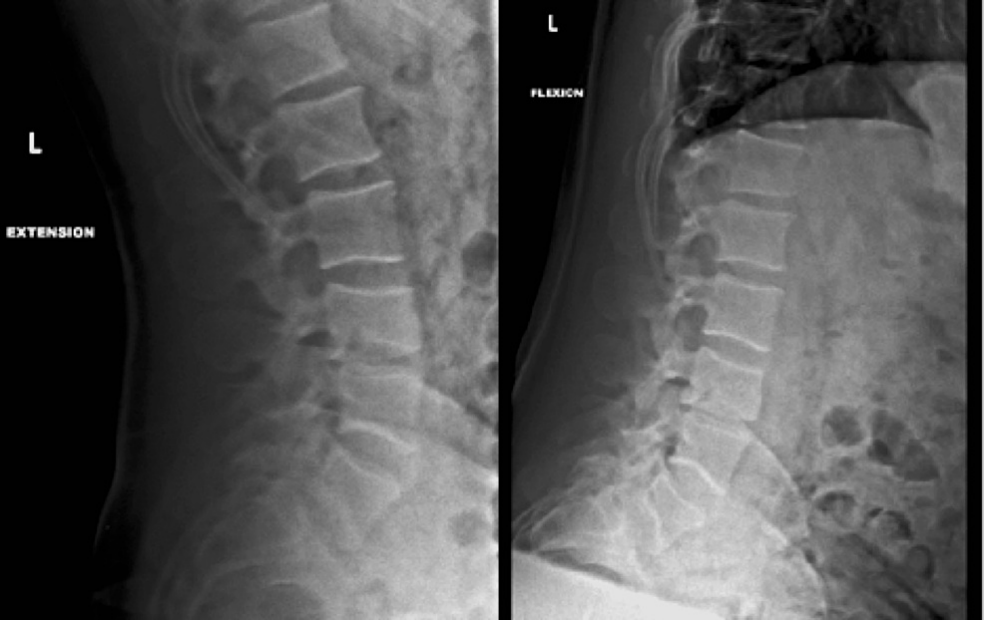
Follow-up flexion and extension X-rays 4 months post-injury demonstrating no mechanical instability
